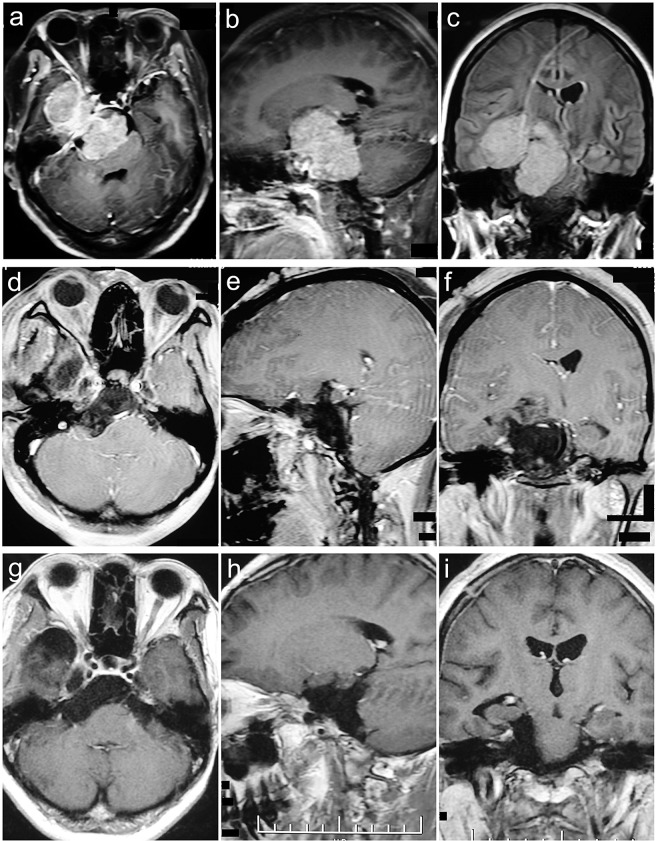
Sphenopetroclival subtype II case. A 41-year-old female presented with epilepsy for 7 months. The preoperative KPS score was 70. She was achieved GTR with the EPTA. The postoperative KPS score was 60. With a follow-up of 30 months, she participated in normal activities and had a KPS score of 90 without recurrence. (a–c) Preoperative MRI T1 contrast axial, sagittal, and coronal images. (d–f) Postoperative MRI T1 contrast axial, sagittal, and coronal images. (g–i) Follow-up MRI T1 contrast axial, sagittal, and coronal images.
